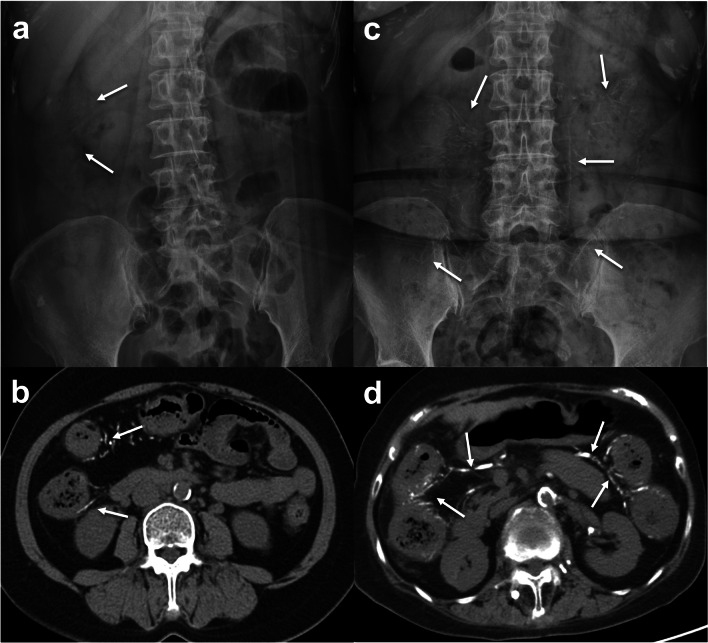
A 58-year-old woman with hepatitis B and chronic renal failure initially presented with mild right abdominal discomfort. a, b Plain radiograph and abdominal CT show curvilinear calcifications (arrows) alongside the distal ascending colon. c, d Mild abdominal pain and intermittent tarry stool noted for 11 years and follow-up radiograph and CT show progression of PC with calcification of superior and inferior mesenteric venous branches (arrows) and colonic wall thickening extending to distal descending colon
